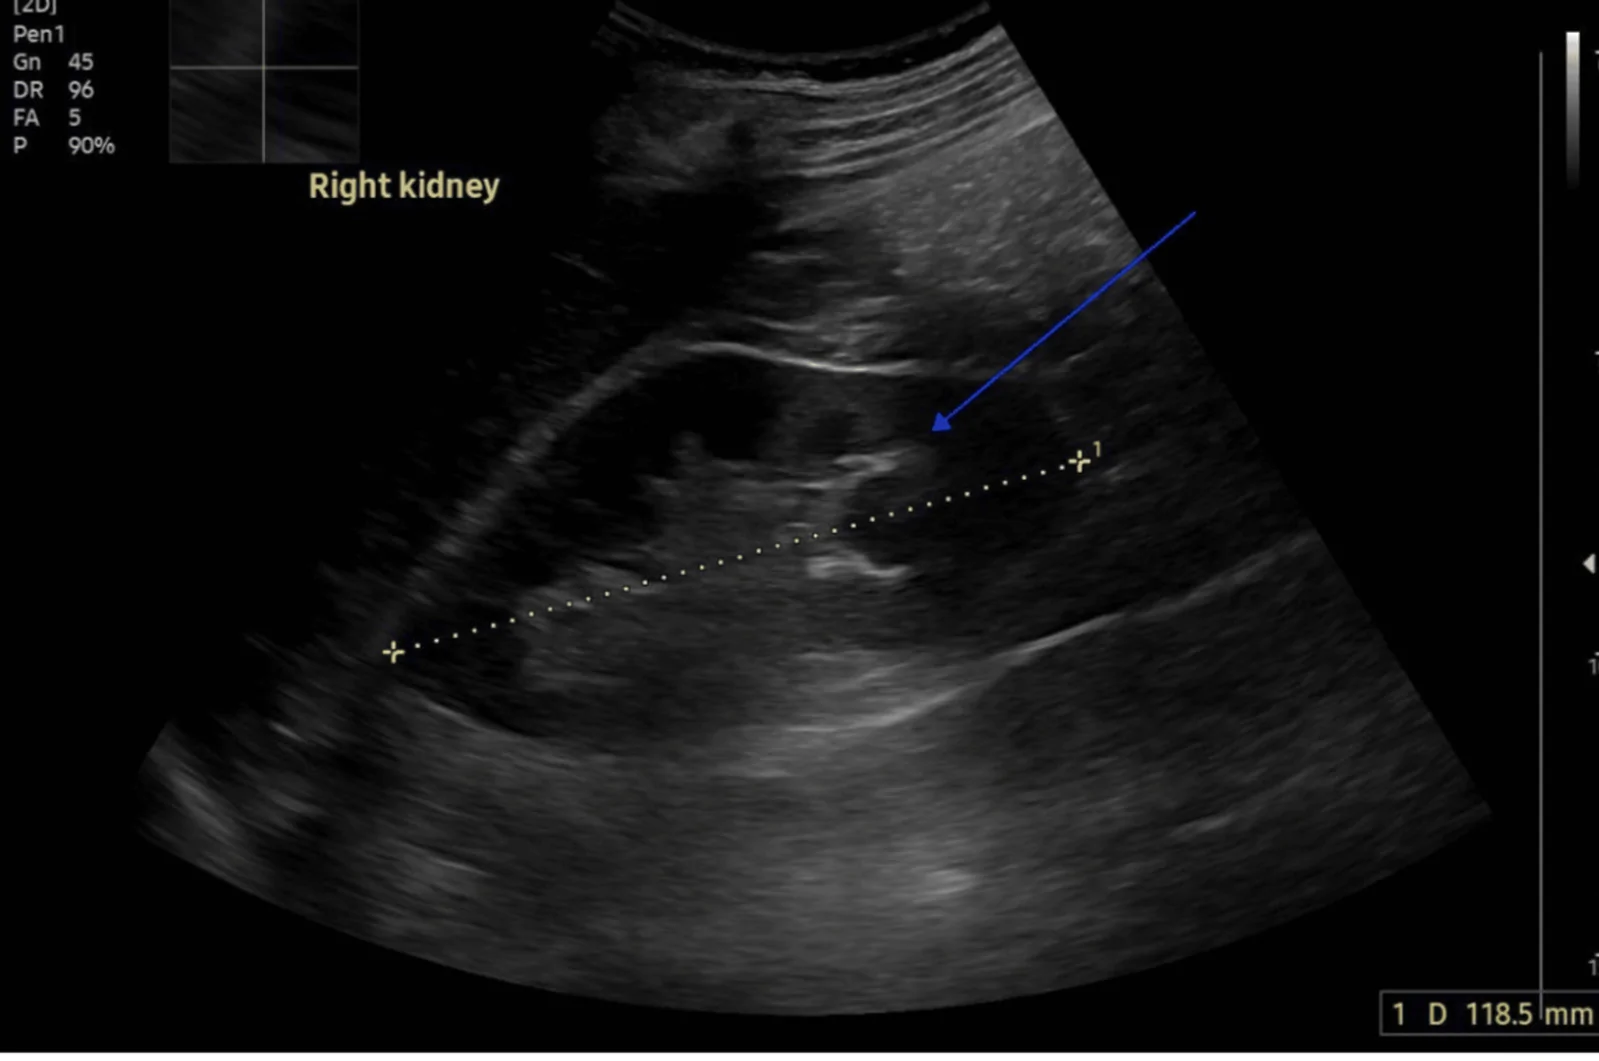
Ultrasound scan showing unobstructed right kidney with normal corticomedullary differentiation (blue arrow)
